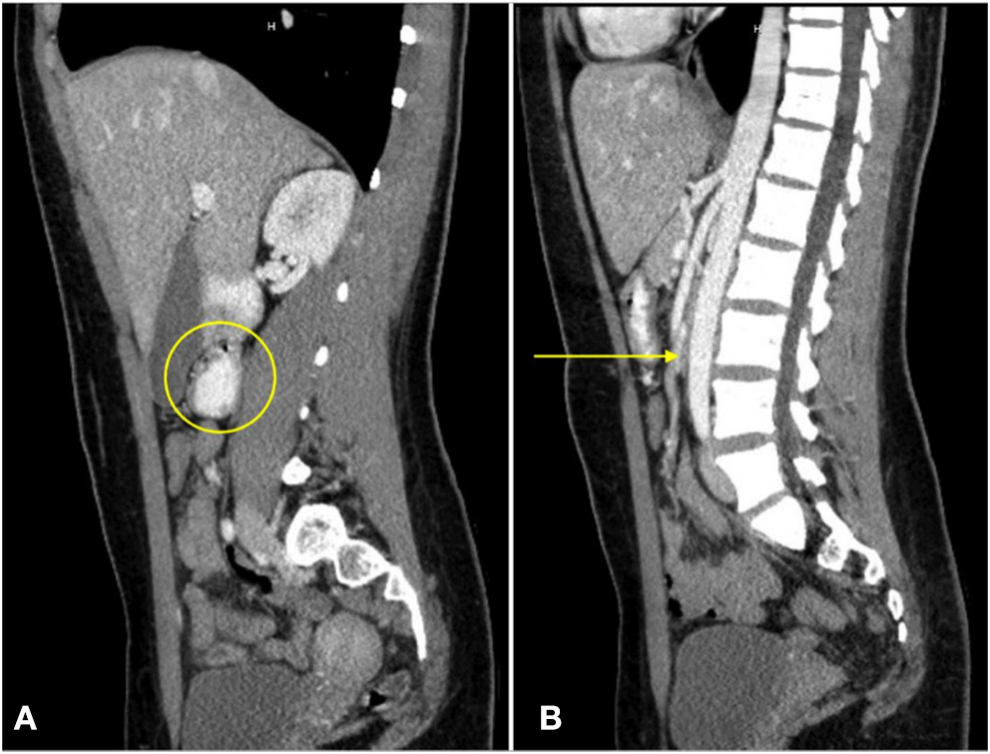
A sagittal CT view of the abdomen with oral contrast reveals dilation of the third portion of the duodenum [(A), circle]. No enteral contrast is seen as the duodenum is compressed between the superior mesenteric artery and the abdominal aorta [(B), arrow].
